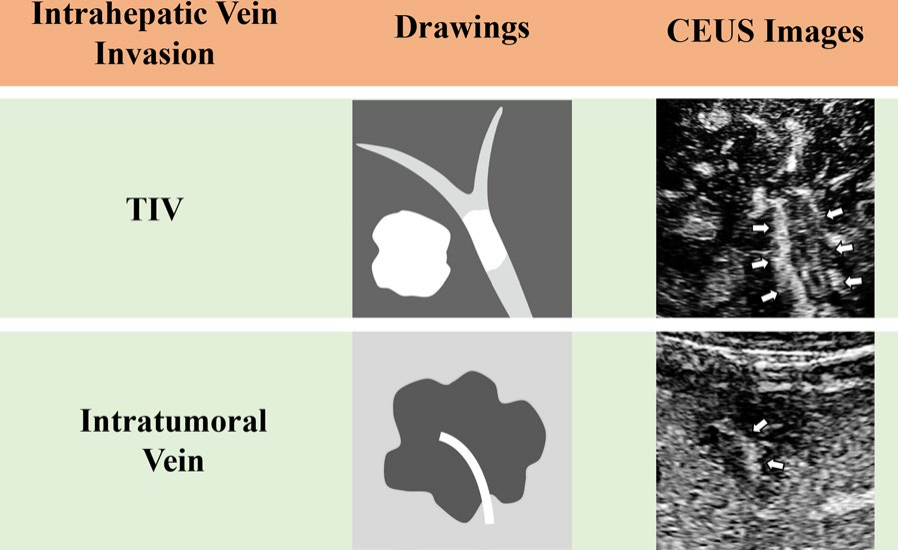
The way of intrahepatic vein invasion. CEUS: Contrast-Enhanced Ultrasound, TIV: Tumor in vein
